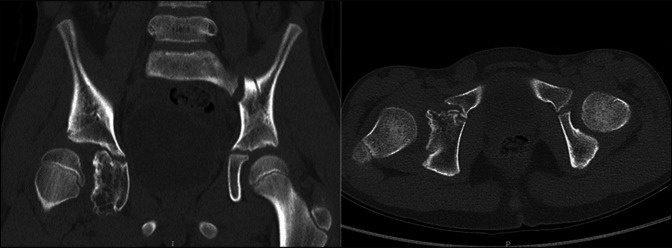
CT imaging demonstrating bony abnormality of inferior acetabulum
